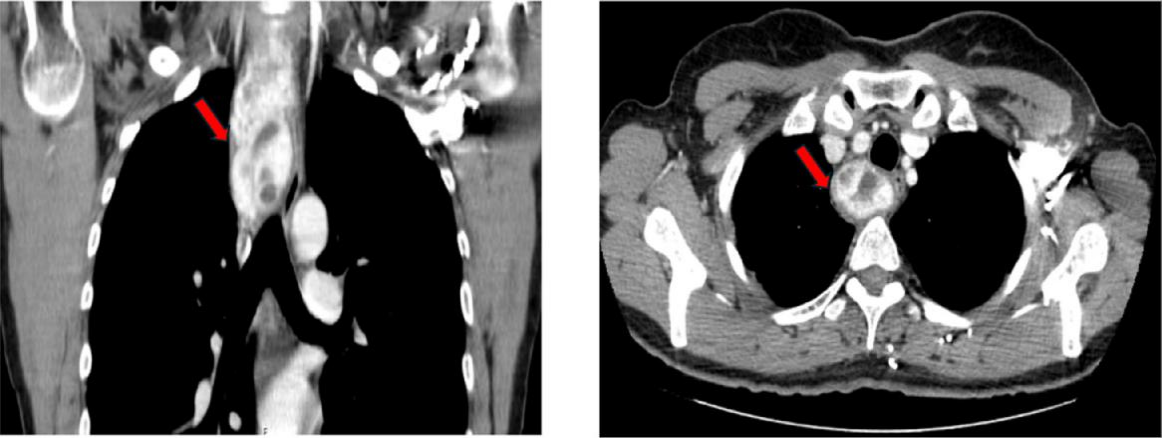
Neck and chest computed tomography. Huge cervicomediastinal mass extending up to the right upper bronchus and contralateral deviation of the trachea and the esophagus.
